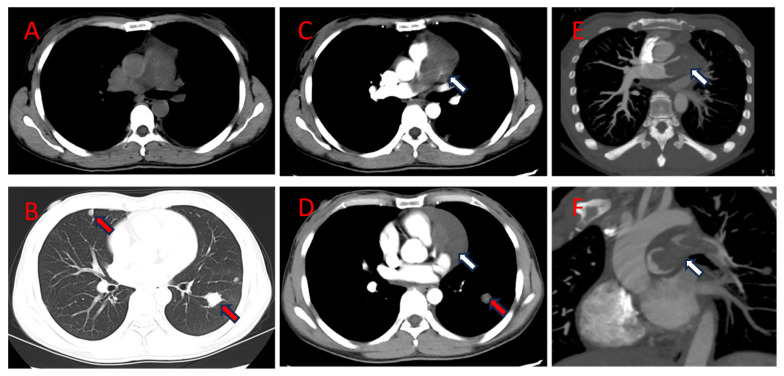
The first chest CT scan of the patient (29 March 2022). Axial CT (A–D) and MIP (E,F) showed an irregular soft tissue mass in the pulmonary artery and anterior mediastinum (white arrow) that had obvious heterogeneous enhancement (B,D). Multiple metastatic tumors in both lungs (red arrow). CT, computed tomography; MIP, maximum intensity projection.
